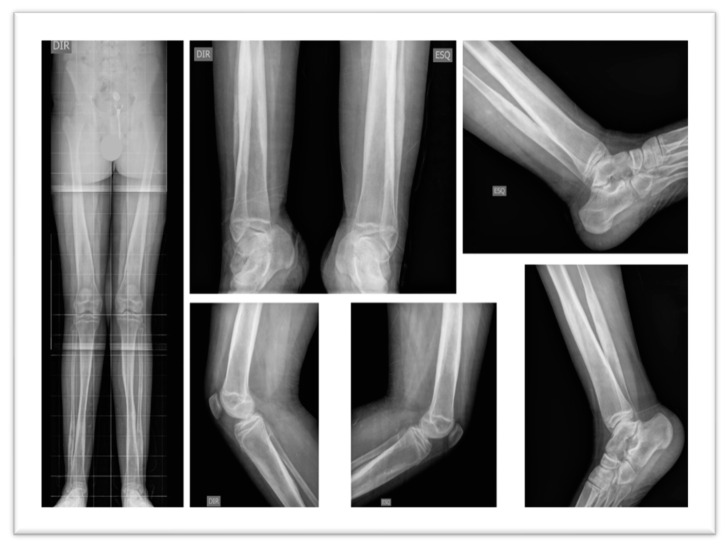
Radiographic Images of the pelvis, lower limbs, knees, and ankles: Bilateral cortical thickening of the diaphysis of the long bones with areas of apparent bilateral and symmetrical hyperostosis (mid/distal femur diaphysis, proximal and distal tibia and fibula).
